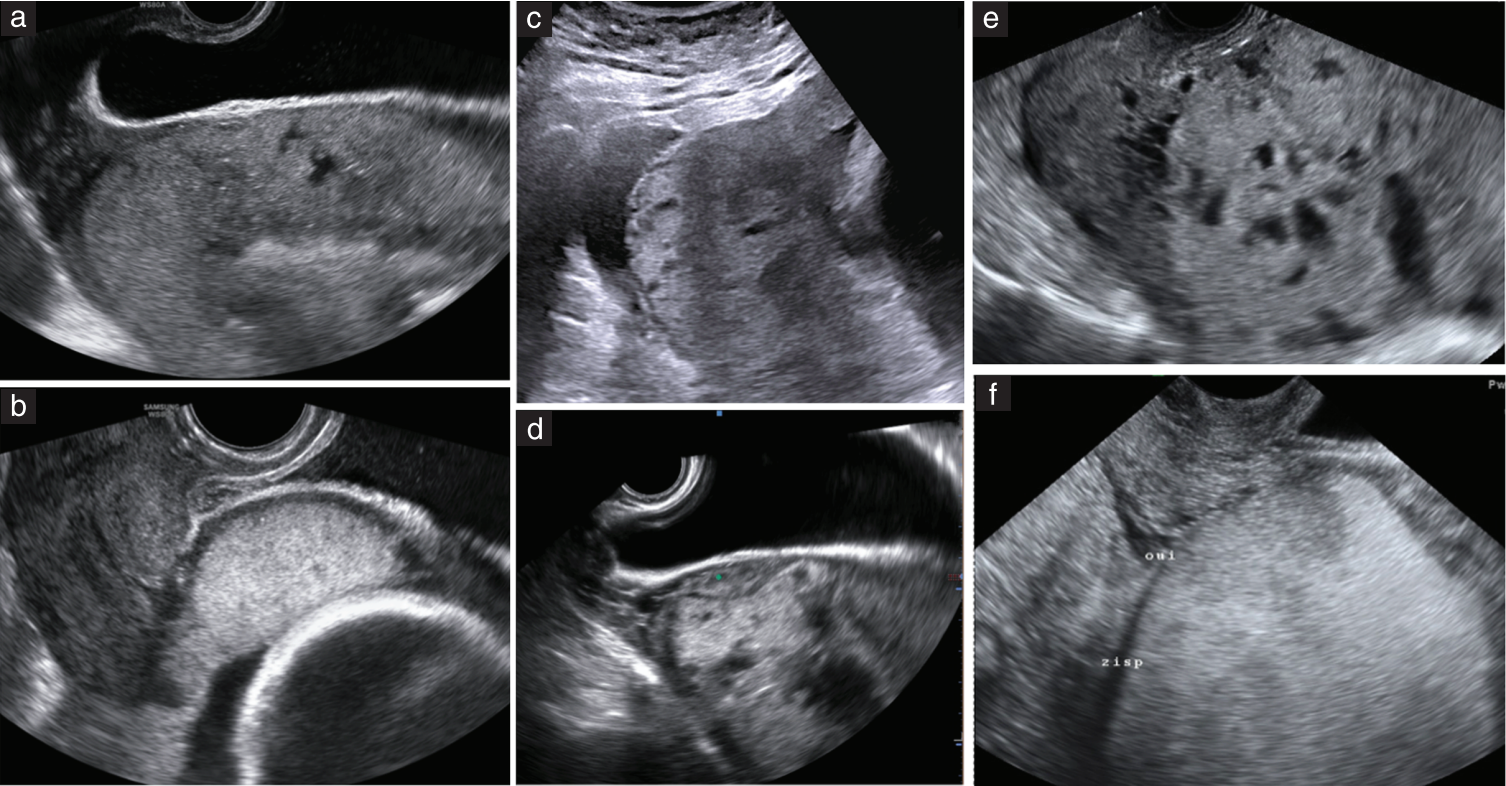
Grayscale ultrasound images obtained at 30–32 weeks' gestation, showing presence (a,c,e) and absence (b,d,f) of sonographic signs of placenta accreta spectrum disorder. (a,b) Obliterated (a) and normal (b) hypoechogenic space between uterus and placenta; (c,d) interrupted (c) and normal (d) hyperechogenic interface between uterine serosa and bladder wall; (e,f) presence (e) and absence (f) of abnormal placental lacunae.
